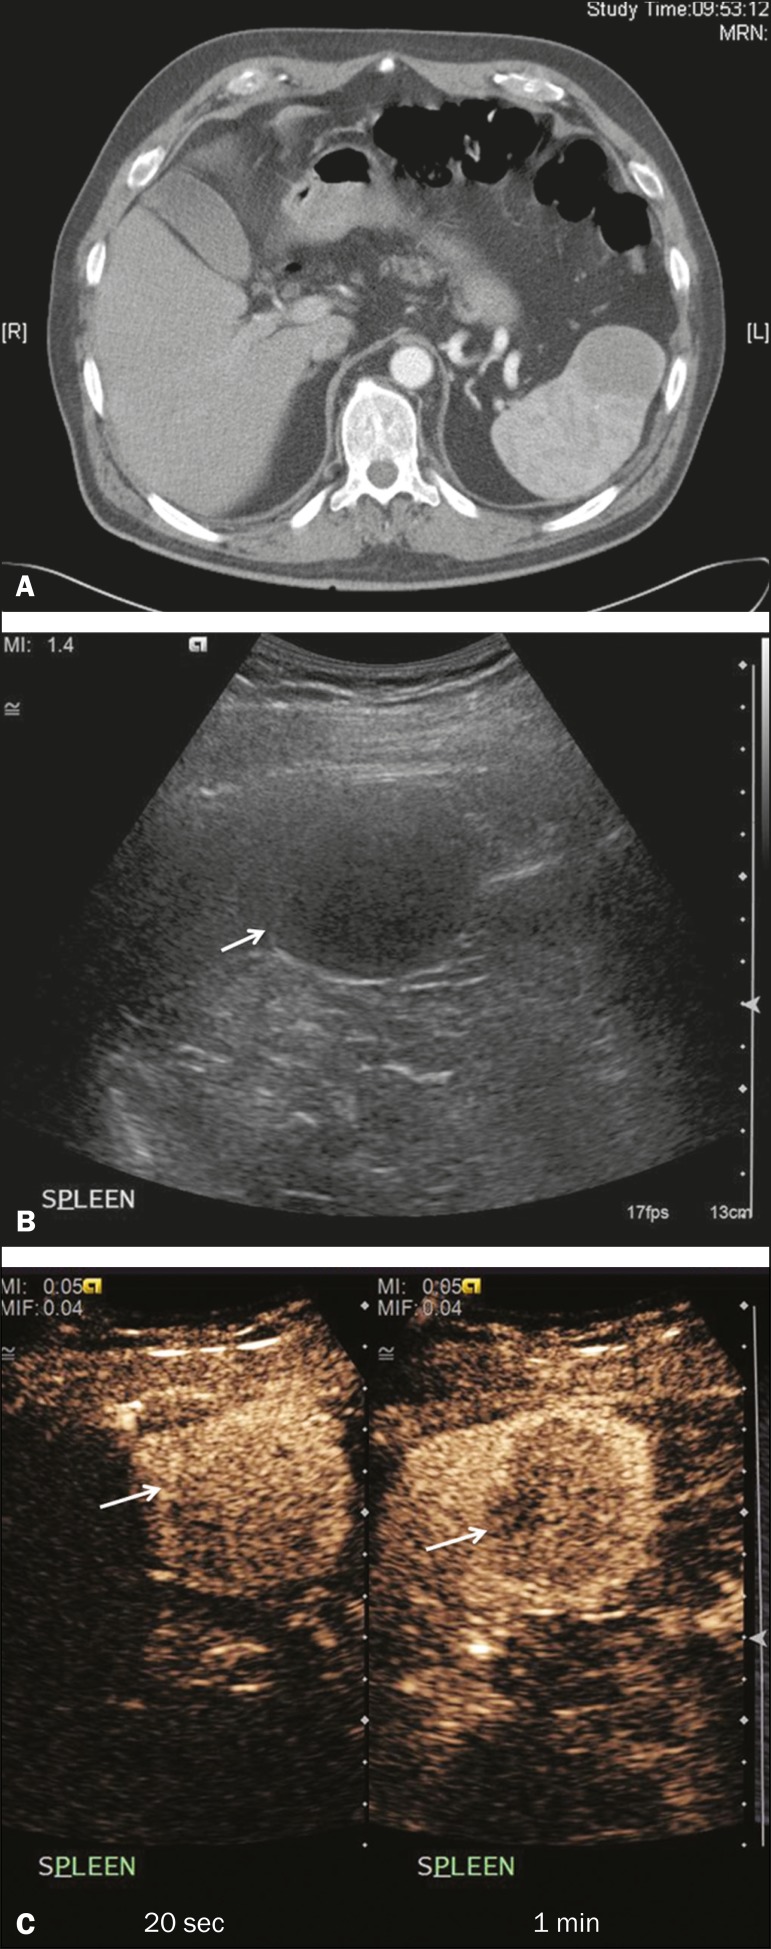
Splenic fibrosis. A: In the routine follow-up CT of a
